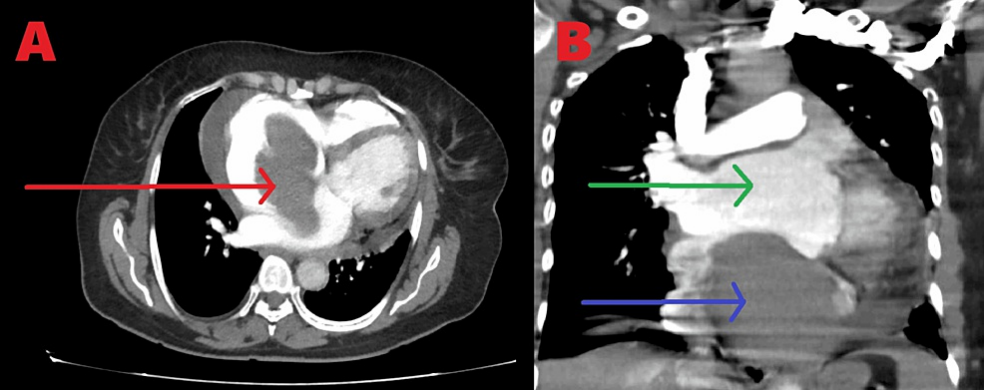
Computed Tomography; A: Red arrow showing a hypodense lesion arising from the atrial septum. B: Green arrow showing the free left atrium and the blue arrow showing the mass in the right atrium.
